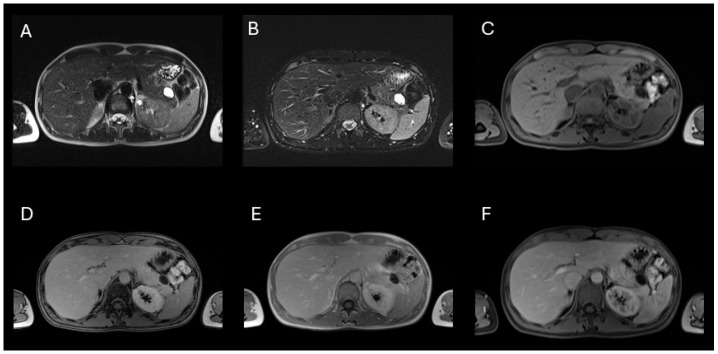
Axial MRI of the abdomen demonstrating a well-circumscribed, multiloculated cystic lesion in the pancreatic tail, consistent with a cystic pancreatic hamartoma. (A) T2-weighted HASTE image shows a hyperintense lesion with internal septations. (B) T2-weighted BLADE image confirms fluid content and sharper margin delineation. (C) Axial T1-weighted VIBE image pre-contrast shows a hypointense lesion. (D,E) In-phase and out-of-phase images reveal no signal drop out, excluding intralesional fat. (F) Portal venous phase post-contrast T1-weighted VIBE image shows no appreciable enhancement of the lesion, with preserved background pancreatic enhancement. The imaging findings are consistent with a benign cystic lesion, and no features suggest malignancy or ductal communication.
